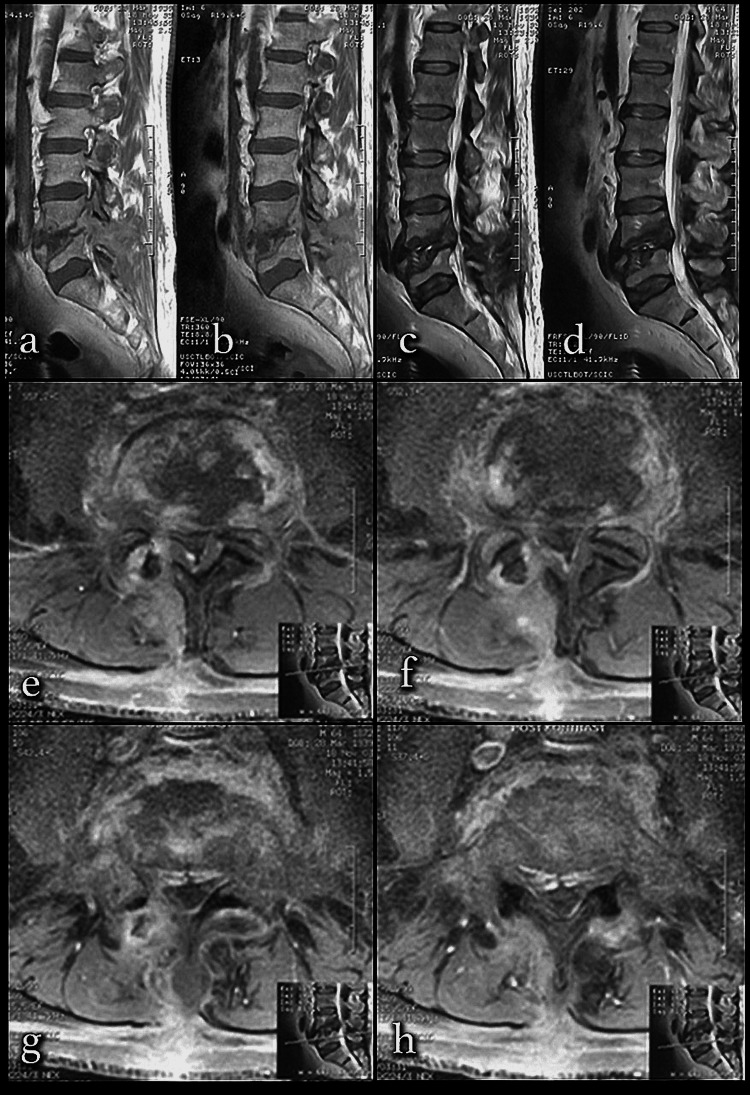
Case of L4-5 tuberculosis after microdiscectomy Destruction of the upper and lower endplates starting from the disk distance a, b, c, and d) T2-weighted sagittal MRI image. The infection, which started at the disc space, caused granuloma and destructed the lower and upper endplates. e and f) T2-weighted axial MRI images: left L4 hemilaminectomy area is seen. g and h) Contrast axial MRI images show destruction of the L4 endplate starting from disc space MRI: magnetic resonance imaging
